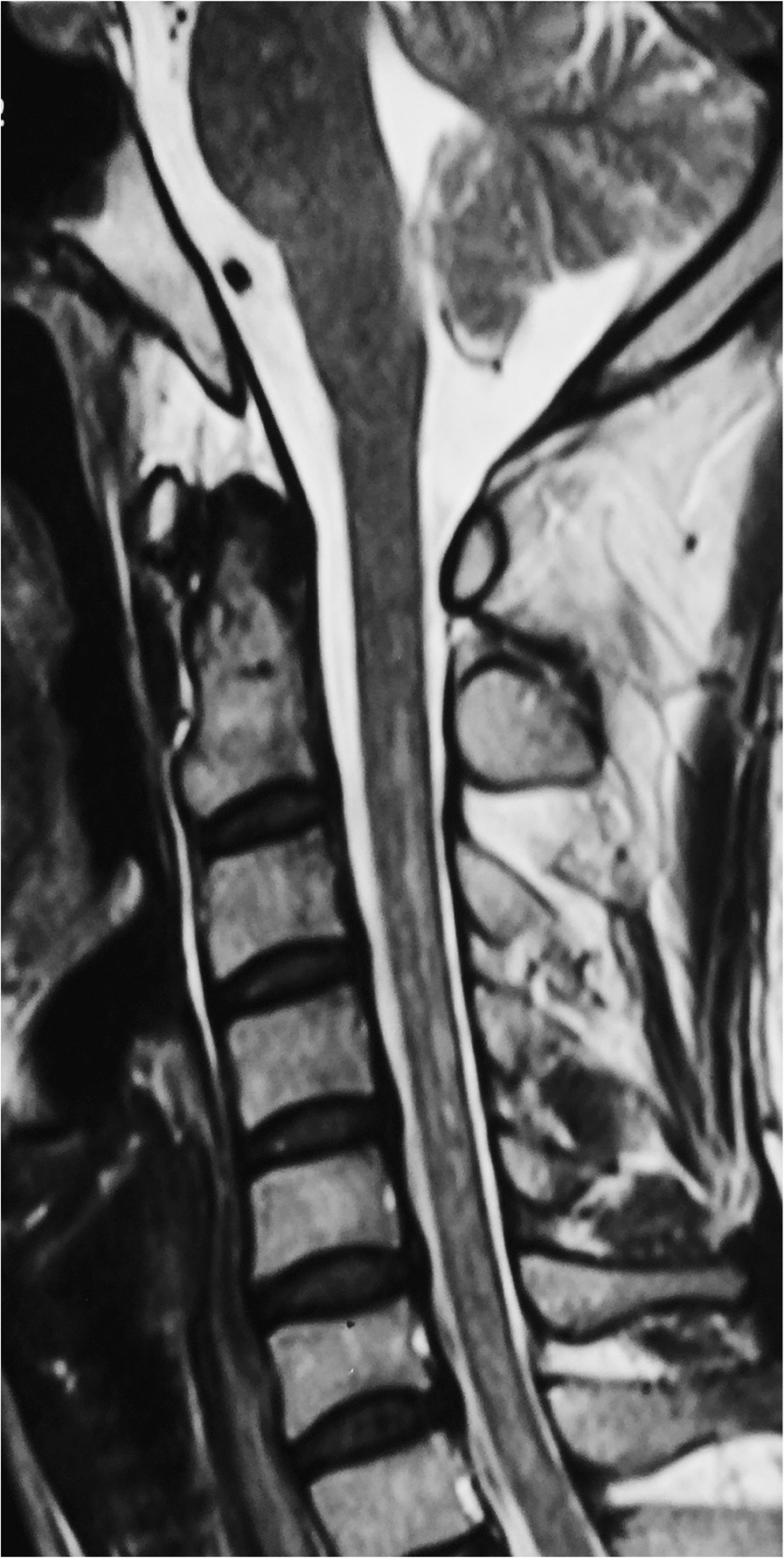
Sagittal T2 sequence MRI showing patchy hyperintensities throughout the cervical spinal cord
